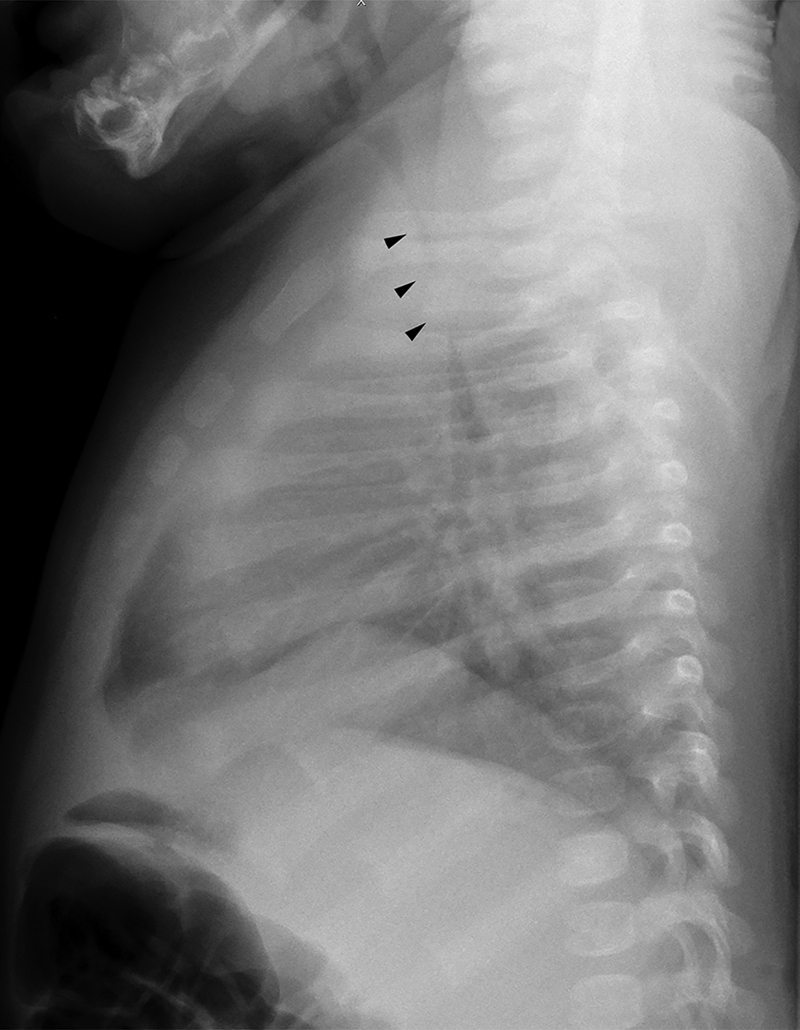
Lateral chest radiograph show tracheal narrowing (arrow heads).
